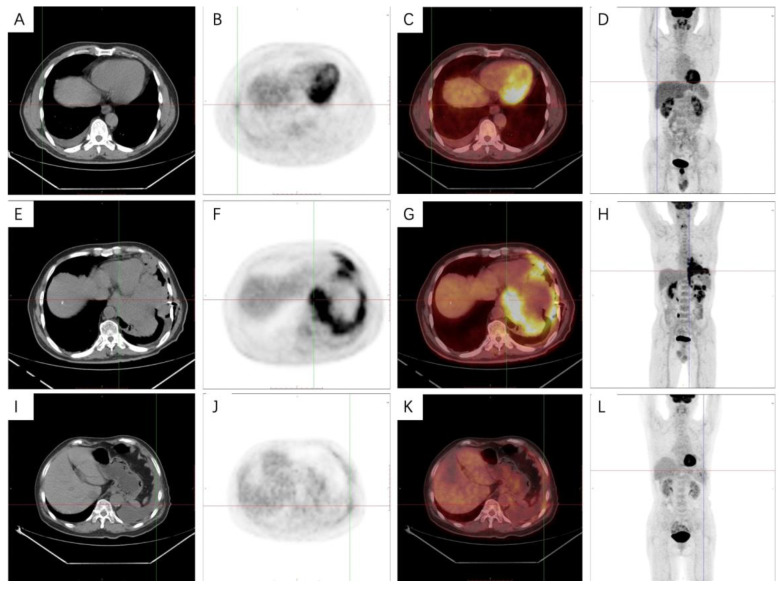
Positron emission tomography-computed tomography images in malignant pleural mesothelioma patients. (A–D) Epithelioid: Focal increased uptake of tracer can be seen in the 6th–7th intercostal pleura on the right side, with a SUVmax of 2.5 and SUVmax of 2.6 on delayed imaging. (E–H) Sarcomatoid: Multiple nodular thickening could be seen in the left pleura, and the tracer uptake was significantly increased with a SUVmax of 7.9. (I–L) Biphasic: Multiple thickening can be seen in the left pleura, with increased tracer uptake and a SUVmax of 3.3.
